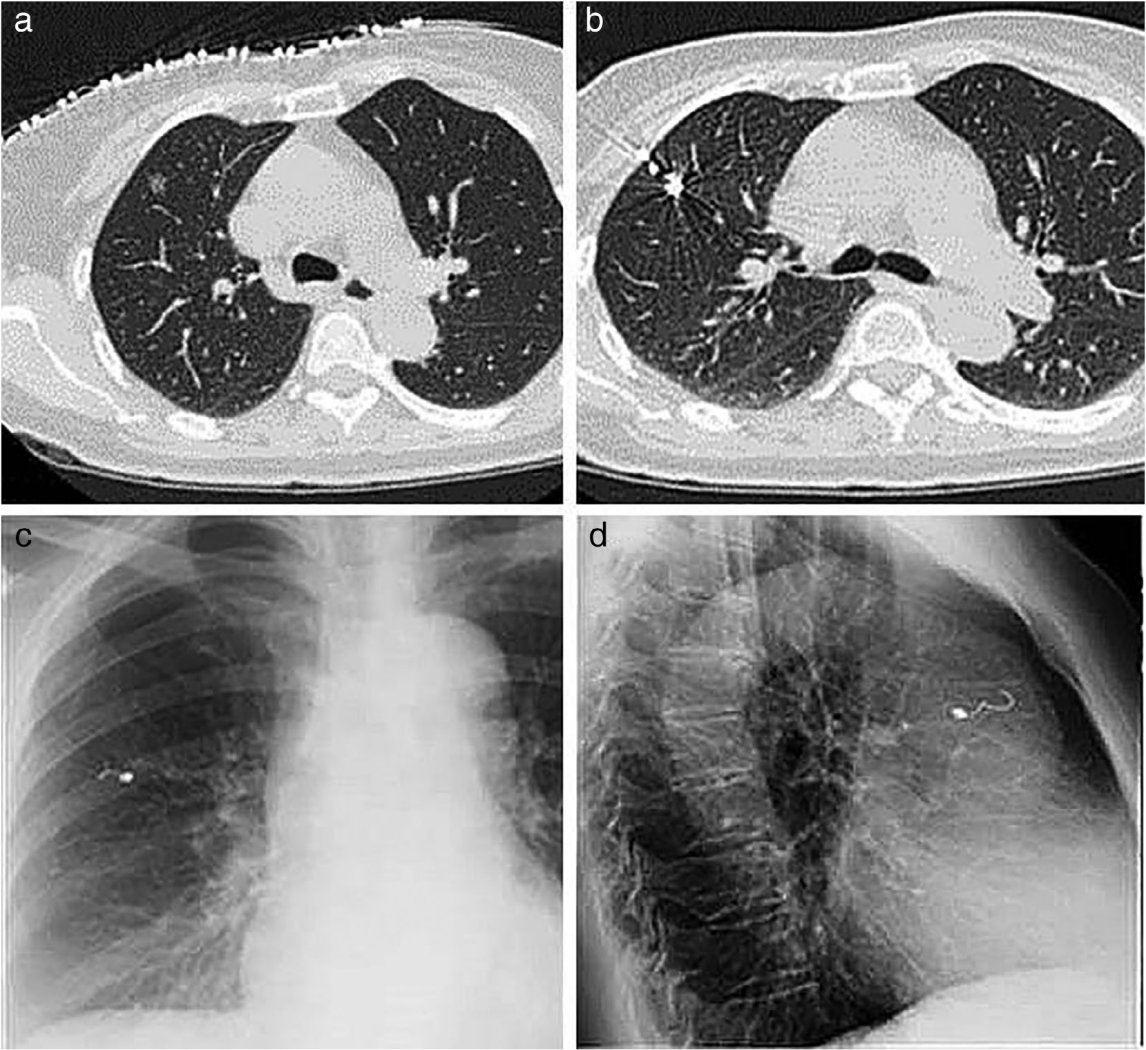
Imaging findings before and after coil positioning operation. (a) CT scan before localization found pulmonary nodules. (b) CT scan during localization confirmed that the puncture need lewas located in the lung tissue around the lesion. (c, d) chest X‐ray after localization showed the proximal part coiling beyond the parietal pleura and the distal part anchoring in the lung parenchyma.
